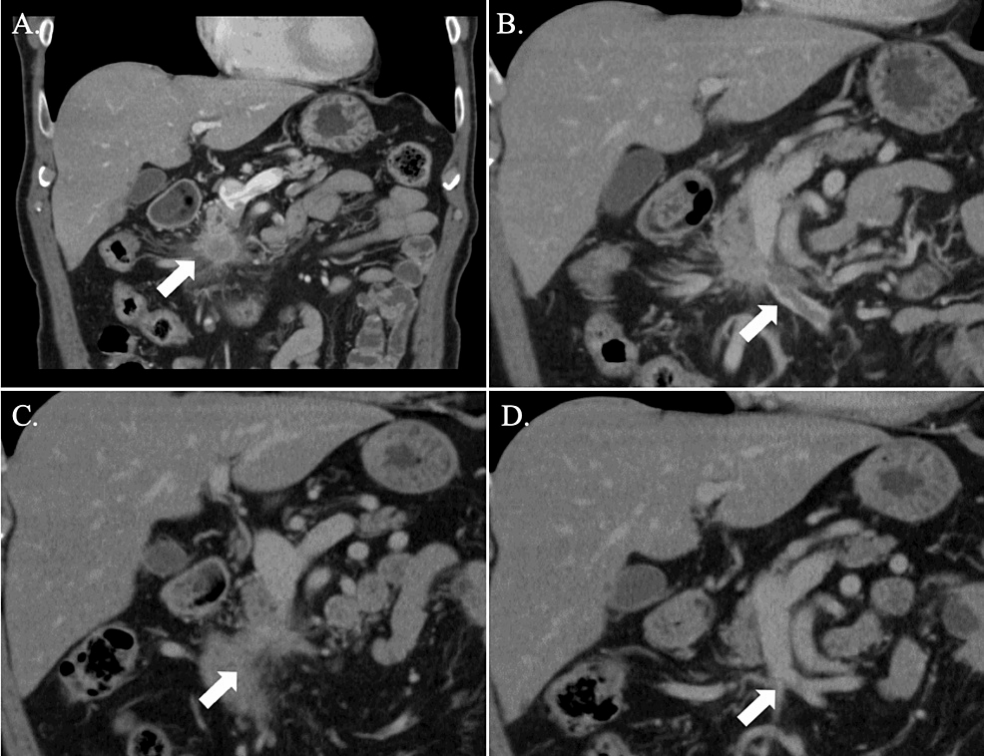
Contrast-enhanced CT images of pancreatic head cancer in the current case before and after the intensive chemotherapy combined with a DOAC. (A) Before the therapy, a nodule with a diameter of around 22 mm and low contrast enhancement was present in the pancreatic head (white arrow). (B) An extensive thrombus was also found in the SMV and J1V (white arrow). (C) After the therapy, main tumor shrank and only soft shadow remained (white arrow). (D) Thrombosis within SMV and J1V had completely disappeared (white arrow). CT; computed tomography, DOAC; direct oral anticoagulant, SMV; superior mesenteric vein, J1V; vein of the first jejunum.
